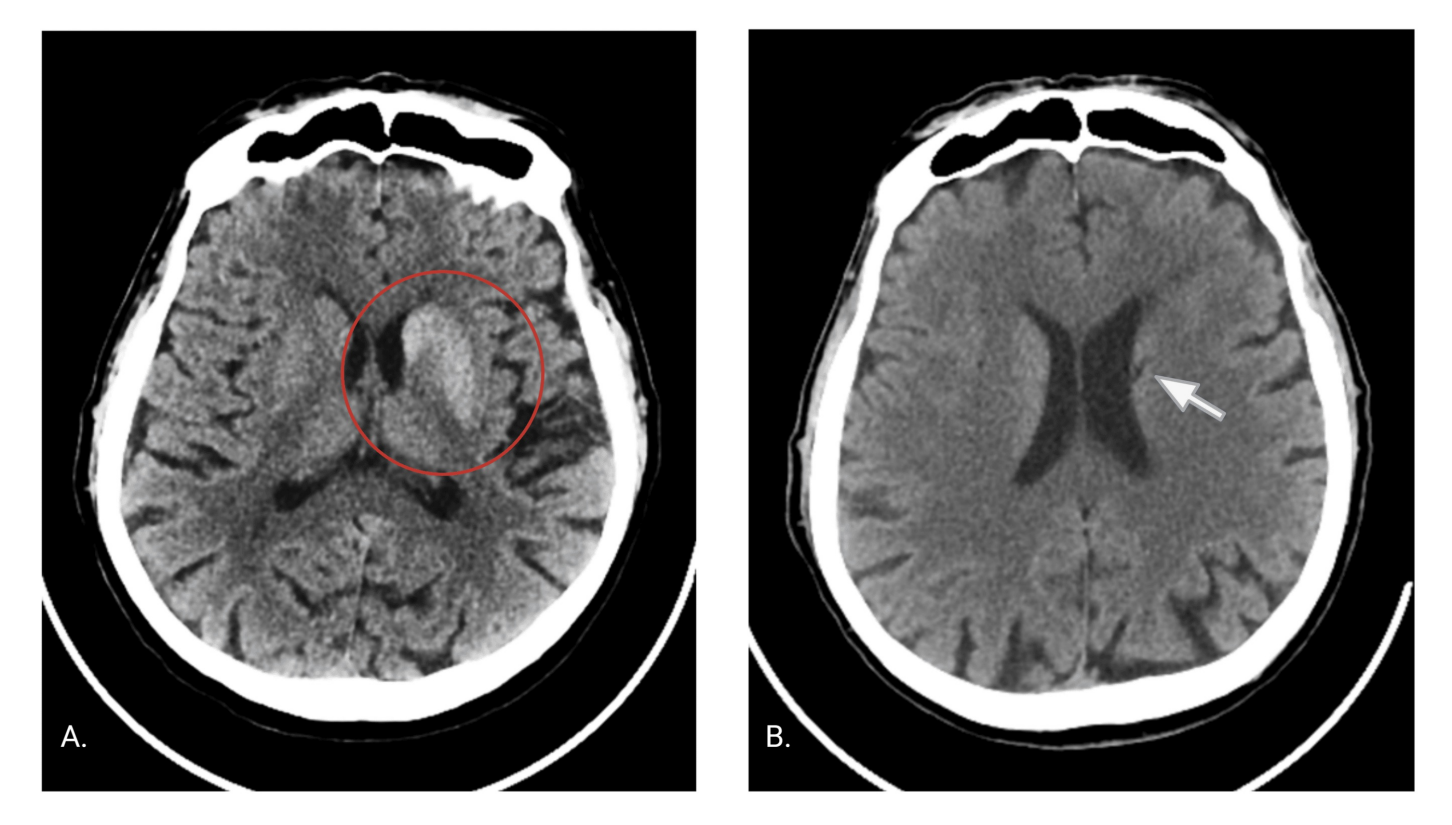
Patient's non-contrast computed tomography (NCCT) of the brain (A) Initial non-contrast CT scan. Marked hyperdensity within the caudate nucleus and the putamen can be observed. (B) Non-contrast CT scan obtained on follow-up. Marked hypodensity within the tail of the caudate nucleus, corresponding to a lacunar stroke.
